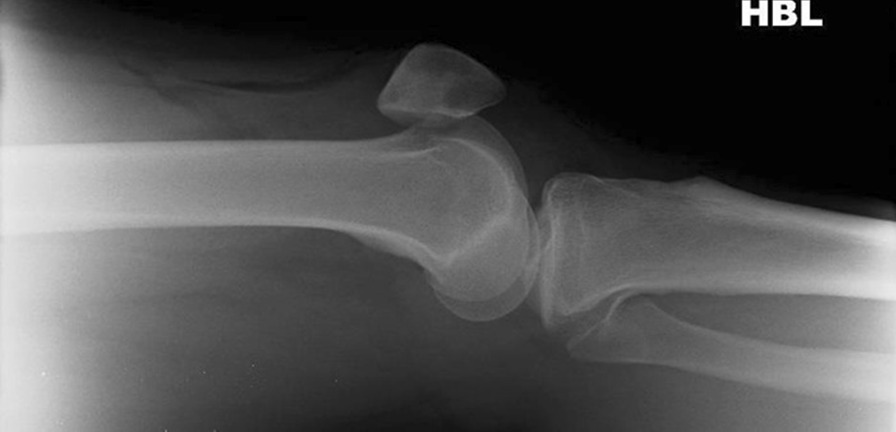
Lateral view knee radiograph upon presentation
